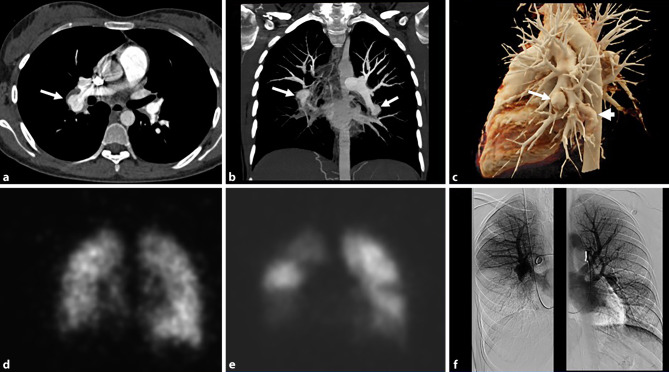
a–c CT pulmonary angiography: a axial reconstruction showing an aneurysm of the right lower lobar artery with wall-adherent thrombus (*arrow*). b Coronal maximum intensity projection (MIP) shows bilateral aneurysms or pseudoaneurysms of the right lower pulmonary artery and left lower lobe segmental pulmonary arteries (*arrows*). c 3D-VRT shows pulmonary aneurysm or pseudoaneurysm (*arrow*) and a varicose aneurysm on the left side (*arrowhead*). d–e Ventilation (d)-perfusion (e) scintigraphy. Coronal view scintigraphy shows extensive perfusion defects in both lower lung fields, the right upper lung field and left middle lung field. f Digital subtraction pulmonary angiography shows right pulmonary artery aneurysm with lower lobe perfusion defect and left segmental pulmonary artery aneurysm that corresponded to the results of perfusion scintigraphy
